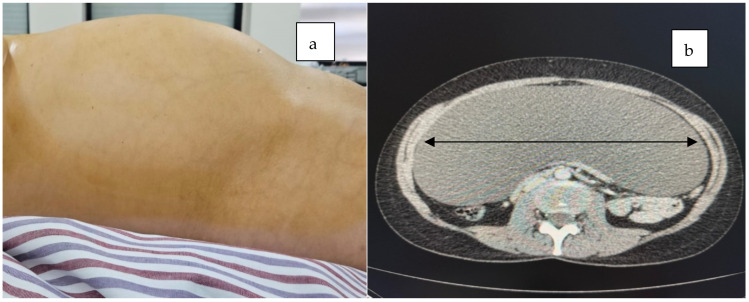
A distended abdomen was observed, predominantly in the middle and lower quadrants (a), and the cross-sectional CT scan revealed a large tumor mass (black double arrow) occupying the entire section (b).
